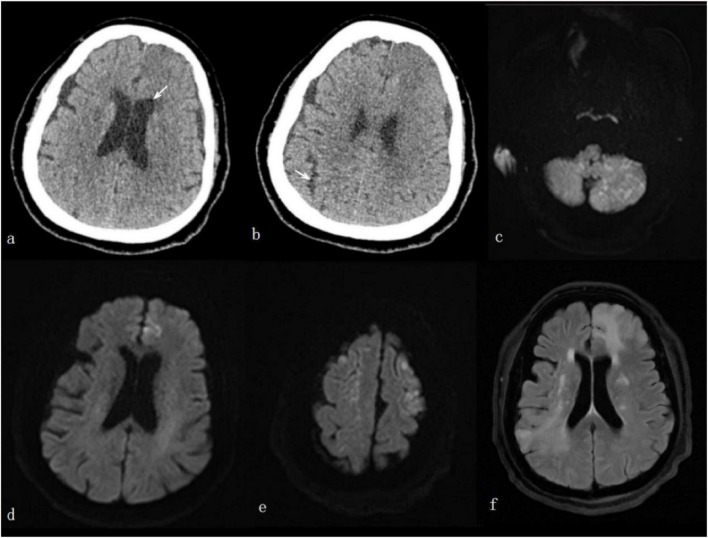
Head CT (a,b) obtained at 4 h after symptom onset demonstrated small air bubbles (arrows) in the anterior horn of the left lateral ventricle and the right cerebral sulci(arrows). Initial diffusion-weighted imaging (DWI) (c–e) showed multiple restricted diffusion lesions in the bilateral frontal-parietal lobes and cerebellar hemispheres; and follow-up brain FLAIR sequence (f) on the 9th day of hospitalization demonstrated that the lesions had fused and enlarged compared to the previous imaging.
